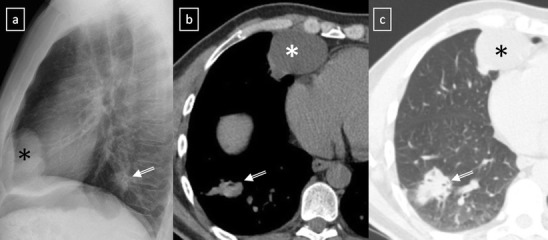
a Lateral chest radiograph of a 58-year-old smoker man allergic to iodine shows a well-defined mass (*) in the cardiophrenic space and a nodular lung opacity (*open arrow*) in the lower lung parenchyma. b, c Non-contrast-enhanced CT scan confirms the presence of a fluid-attenuation mass (*) in the right cardiophrenic angle (pericardial cyst) and demonstrates a suspicious lung opacity (*open arrows*) in the right lower lobe of the lung (squamous-cell carcinoma)
